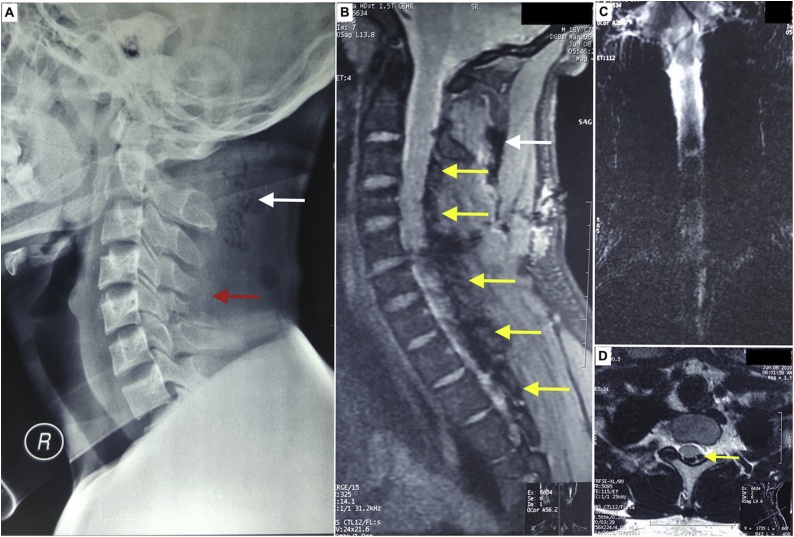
Radiological examinations A: Lateral cervical x-ray showing fractures of C4 and C5 spinous process (red arrow) and subcutaneous emphysema (white arrow). B: Sagital T1 cervical MRI showing subcutaneous emphysema anterior to the splenous capitis muscle (white arrow), disruption of soft tissue along the path of the blade that transected the spinal cord between C5-C6 and pneumorrhachis extending from C2–T1 (yellow arrows). C: Coronal T2 cervical MRI showing impaired cerebrospinal fluid flow caudal to the level of C2. D: Axial T1 cervical spine showing compression of the spinal cord due to trapped air (yellow arrow) within the spinal canal.
